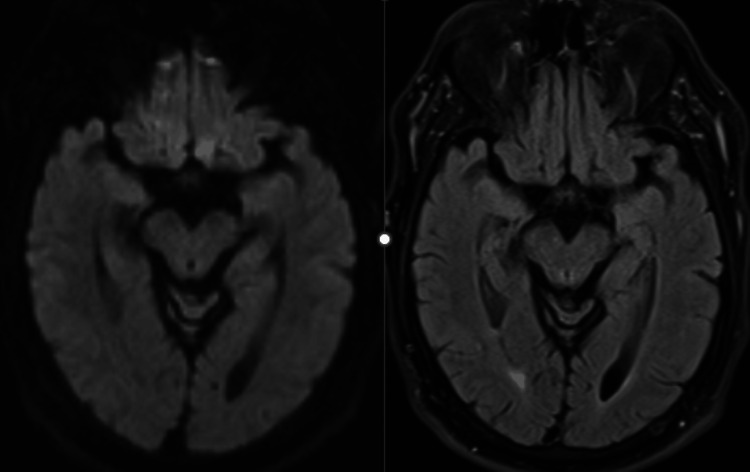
MRI DWI (right) and FLAIR (left) sequences of the midbrain showed no abnormality DWI: diffusion-weighted imaging; FLAIR: fluid-attenuated inversion recovery; MRI: magnetic resonance imaging
